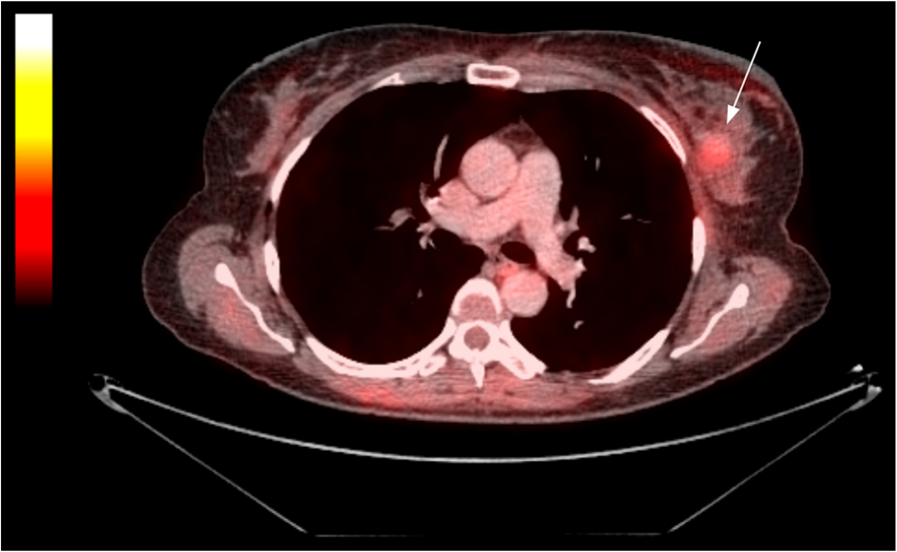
PET/CT showing FDG-avid lesion 19 × 14 mm in the left mammary gland
